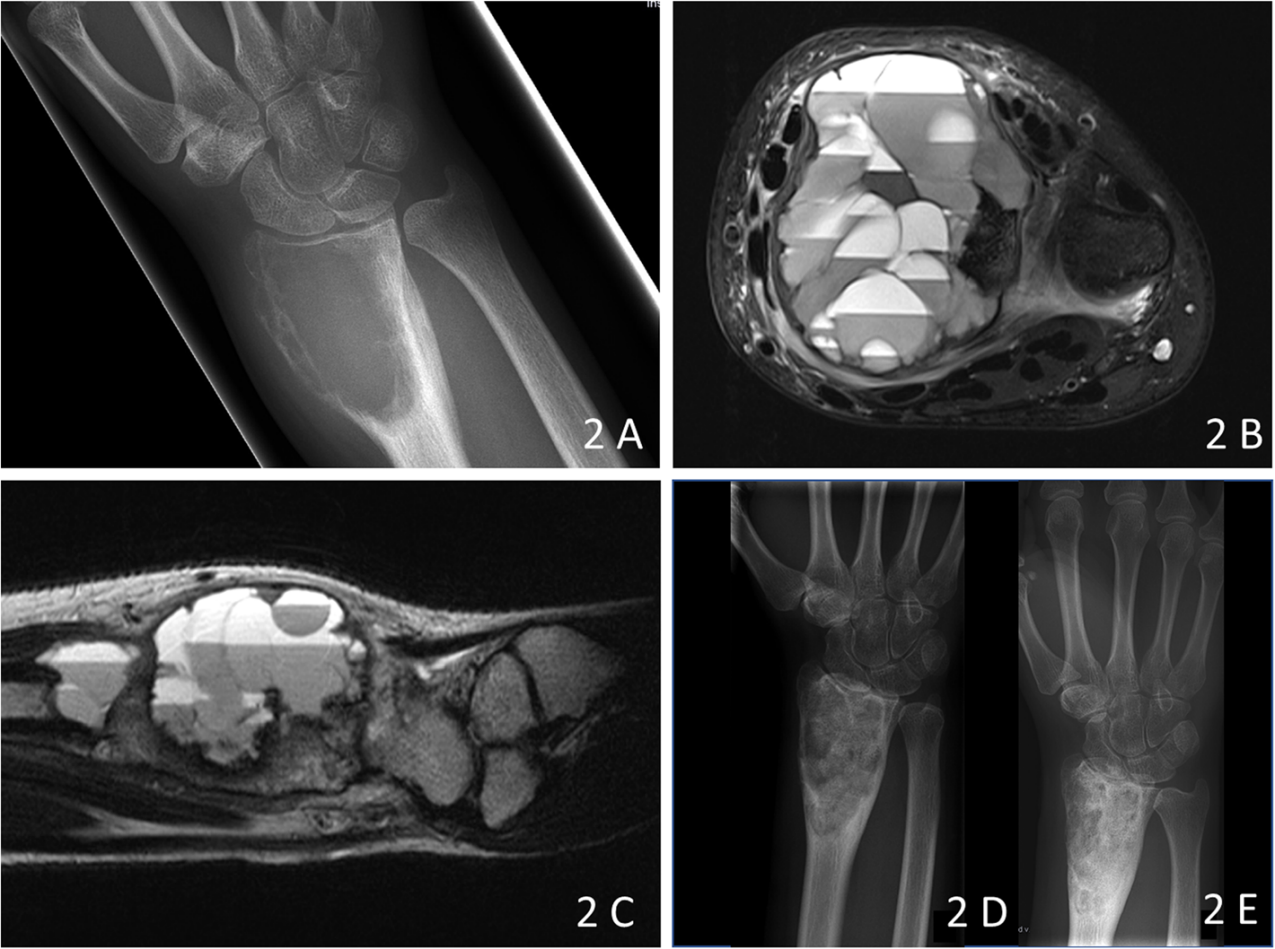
a Radiograph of the right distal radius showing the typical metaepiphyseal exentric osteolysis of an ABC of bone. b: The lesions shows typical fluid- fluid levels on T2-weighted TSE imaging with the patient in supine position. c: Local recurrence 3 months after curettage and bone grafting. d, e: Radiographs 2 months (d) and 1 year (e) after surgery and starting of Denosumab therapy
